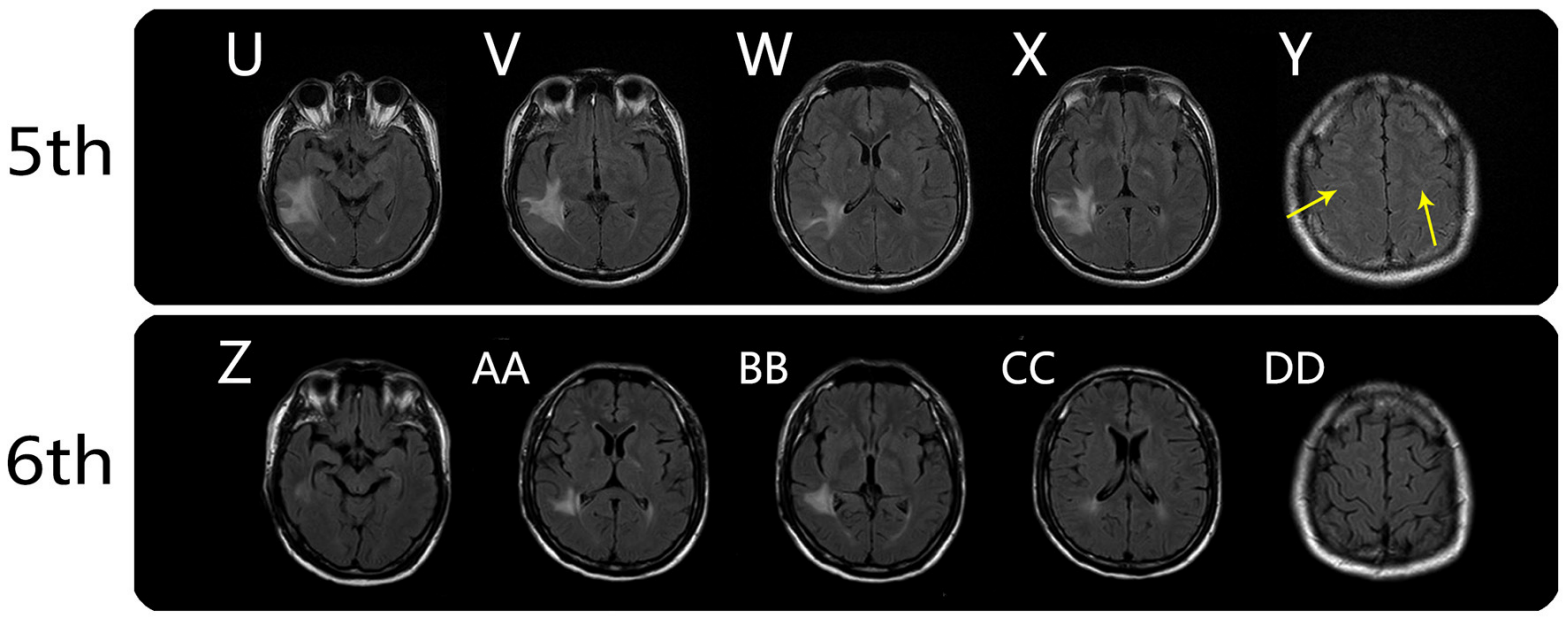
Brain MRI findings from the fifth and sixth MRI scans. Fifth MRI scan (on day 132): axial FLAIR showed persistent meningo-cortical hyperintensity (Y, arrows) and new lesions around the temporal lobe, posterior limb of internal capsule (right), and left trigone of the lateral ventricle (U–X). Sixth MRI scan (on day 148): axial FLAIR showed substantial reductions in the original lesions without new lesion formation (Z, AA, BB, and CC), and the meningo-cortical FLAIR hyperintensity disappeared (DD).
